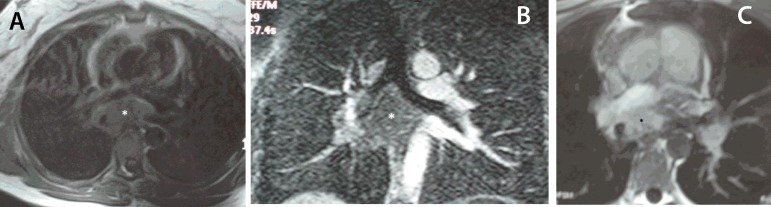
A: Axial T1-weighted image. The mass is almost isointense to the thoracic
